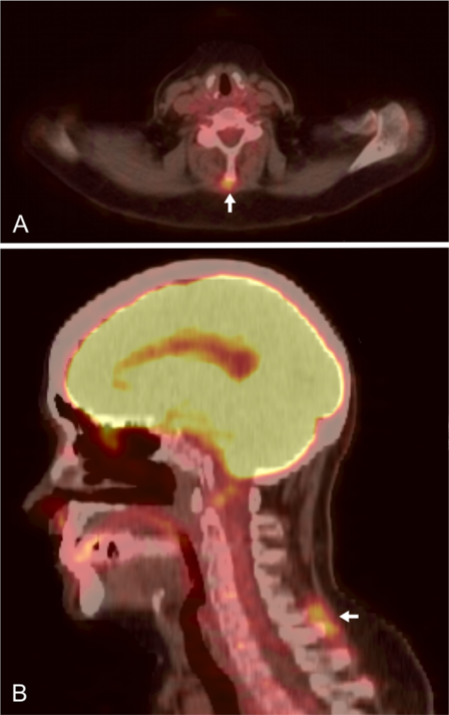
FDG uptake in the cervical interspinous spaces of a patient with polymyalgia rheumatica. (A) Axial PET/CT section at the level of the 5^th^ cervical vertebra; (B) sagittal PET/CT image showing bursitis (arrows) between 5^th^ and 6^th^ cervical vertebra. FDG, 18 F-fluorodeoxyglucose; PET/CT, positron emission tomography integrated with computed tomography.
